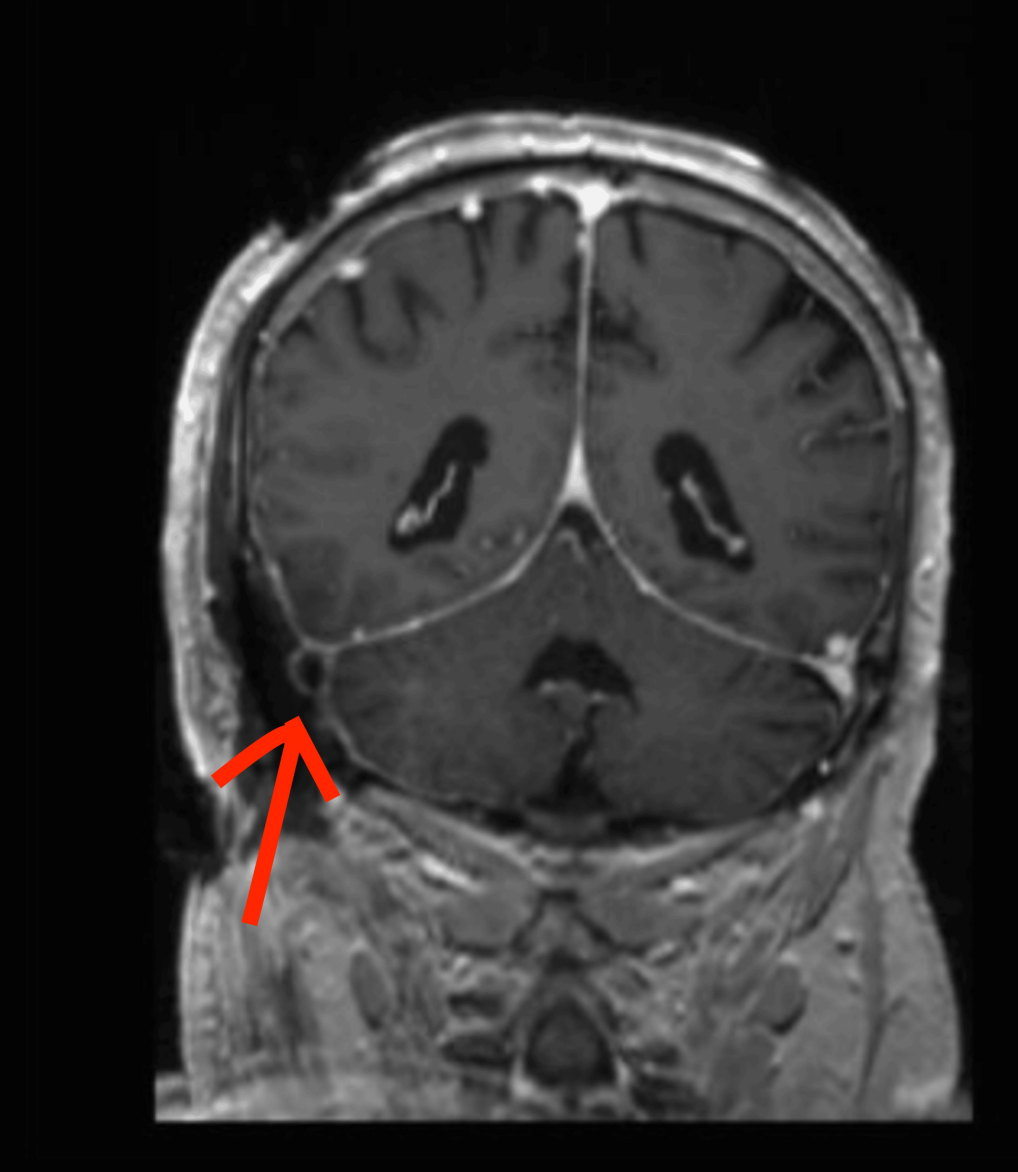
Post-operative MRI with and without IV contrast Acute thrombosis of the right transverse/sigmoid sinus seen on post-op MRI.
